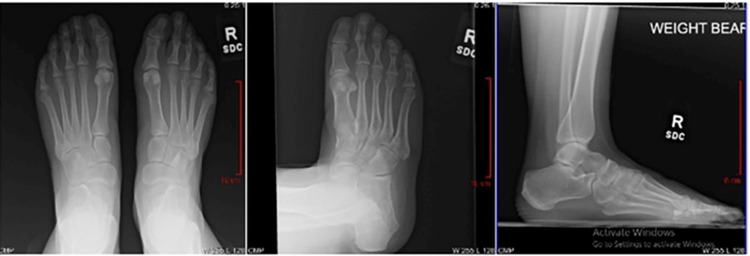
Example of mock patient scenario (Case 1) provided to surgeons; Pre-operative radiographs demonstrating 2nd metatarsophalangeal joint dislocation with metatarsal head overload.
